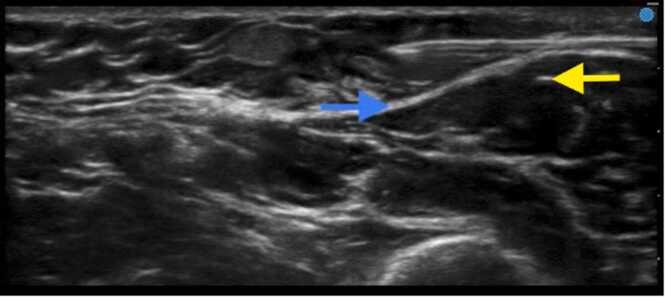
Ultrasound-guided intercostobrachial and medial brachial cutaneous nerve blocks. Fascia overlying the biceps brachii muscle depicted as a blue arrow and implant as a yellow arrow. Depth markers are seen on the right side of the image (total depth of the ultrasound image is approximately 2.3 cm).
